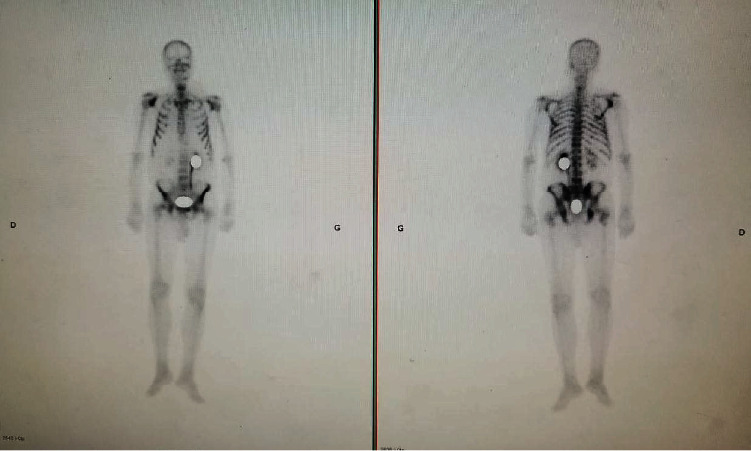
Bone scintigraphy showing several metastatic lesions, including in the lumbar spine.
